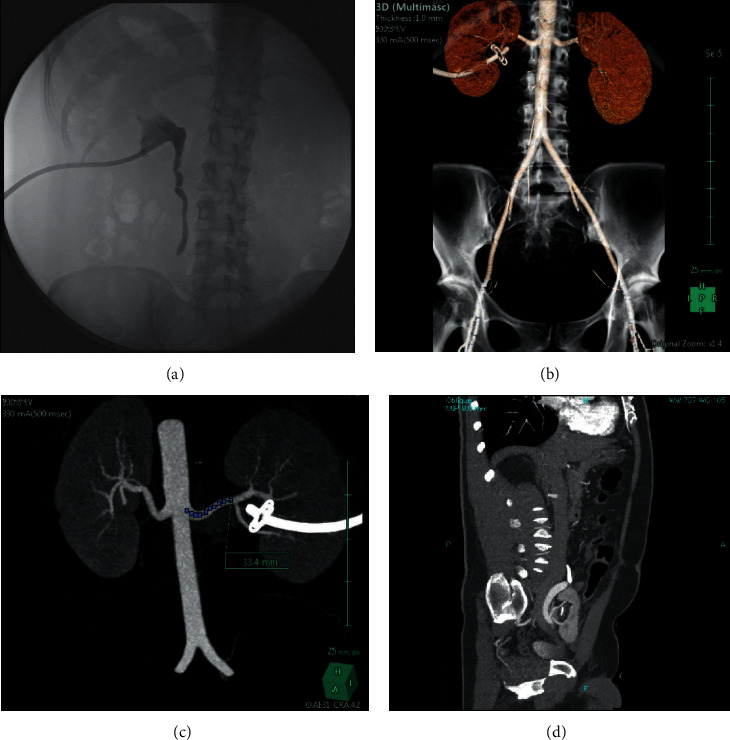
(a). Antegrade pyelography showing ureteral stop secondary to an iatrogenic ureteral lesion during open surgery. (b) and (c). Three-dimensional computed tomography reconstruction of the artery Ao, abdominal aorta. (d) Sagittal CT image showing KAT location and vasculature.
